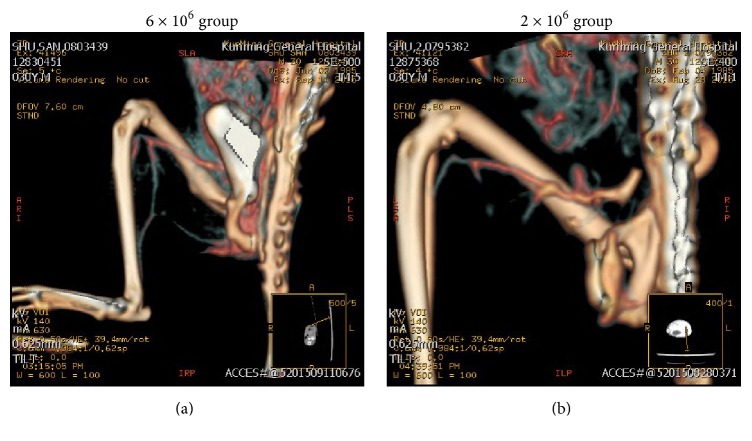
Angiography (arterial CTA). Angiography was performed 21 days postoperatively. (a) The injection of 6 × 10^6^ UC-MSCs significantly promoted angiogenesis. (b) Injection of 2 × 10^6^ UC-MSCs showed less effectiveness.
